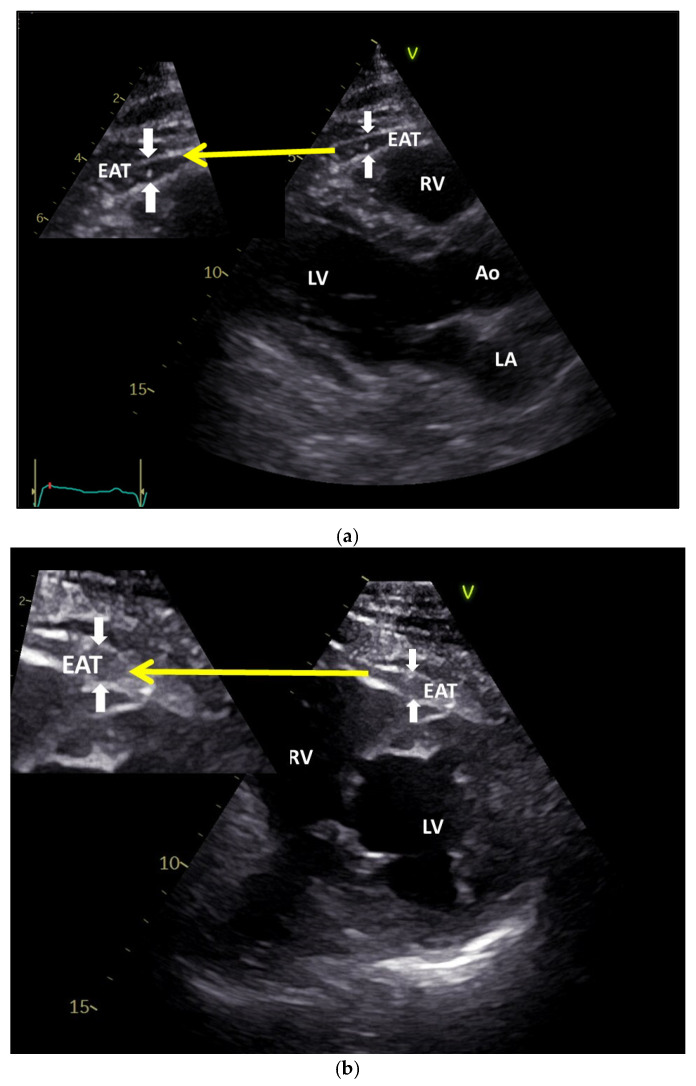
Epicardial fat by transthoracic echocardiography. (a). Epicardial fat thickness at parasternal long axis (the distance from outer side of myocardium to pericardium). (b). Epicardial fat by transthoracic echocardiography. Epicardial fat thickness at parasternal short axis (the distance from outer side of myocardium to pericardium). EAT—Epicardial Adipose Tissue; RV—Right Ventricle; LV—Left Ventricle; Ao—Aorta; LA—Left Atrium. White arrows pointing towards the EAT; Yellow arrow pointing towards the magnified view of EAT.
